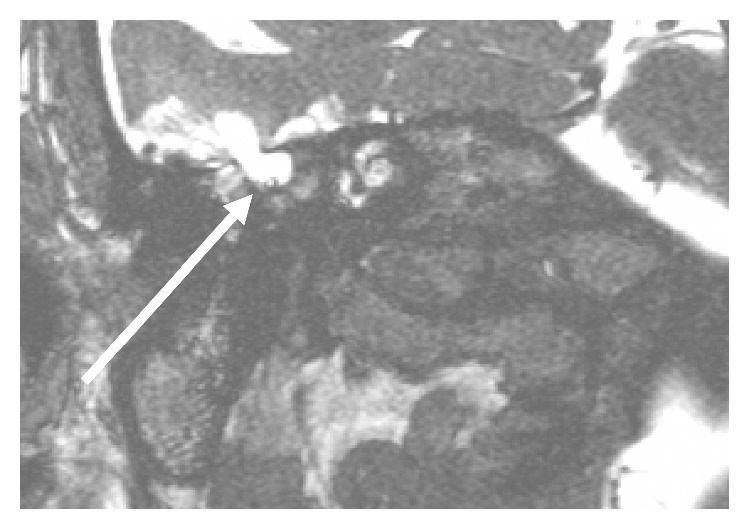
MRI scan with defect and the herniating cyst: MRI diffusion-weighted scan (coronal view).
